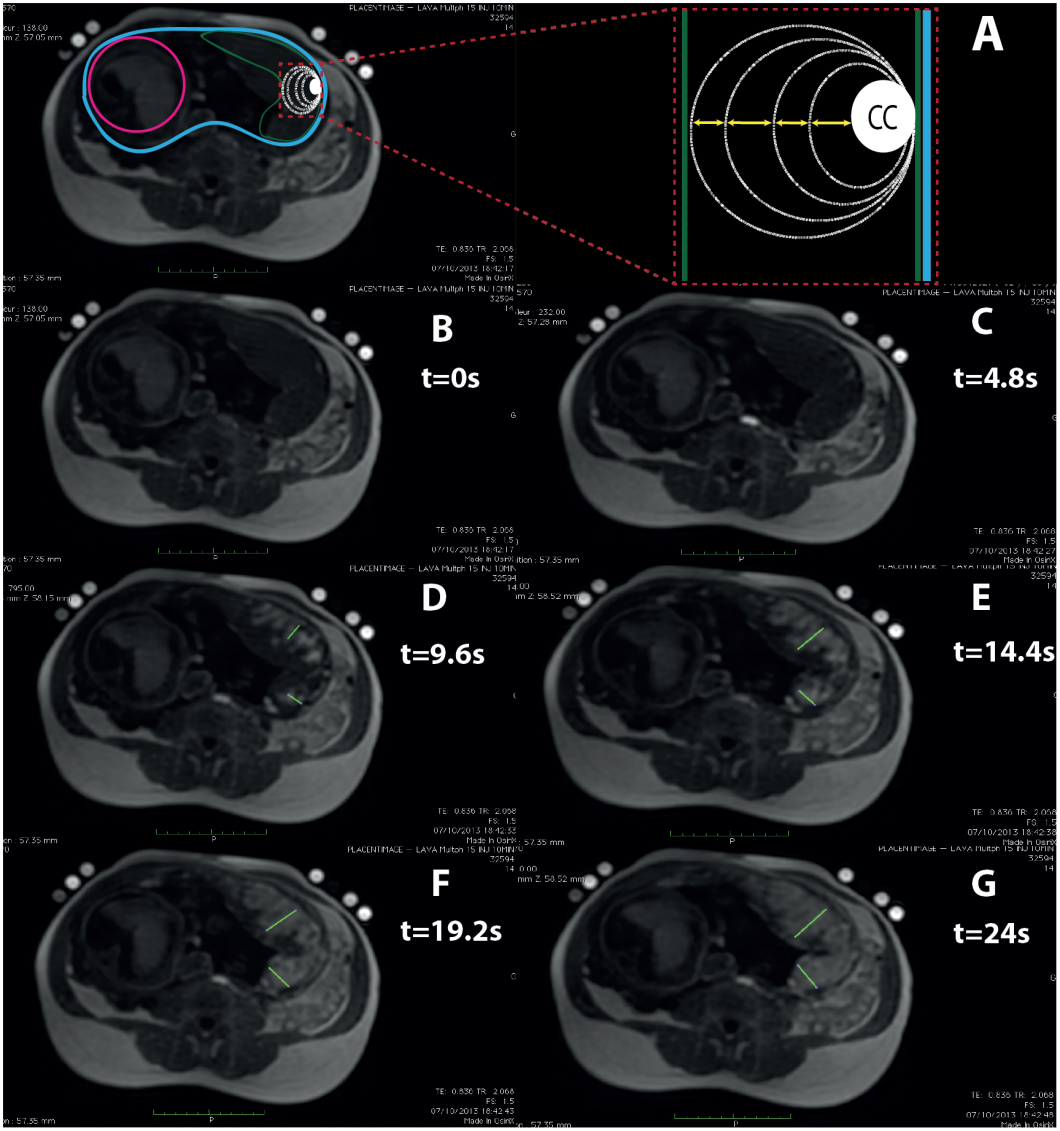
Dynamic placental MRI. A: Placenta (green line), uterus (blue line), fetus (pink line). The intraplacental progression of the contrast-enhanced maternal blood in the IVS was measured (yellow arrows) every Δt in a perpendicular direction between the basal plate and the chorionic plate. B-G: Progression of the gadolinium is measured every 4.8s, from the first MRI picture with gadolinium in the placenta (Fig 6D) in order to avoid calculating the velocity of the maternal blood in the central cavity (CC) of the placentone. The mean flow velocity of maternal blood measured in the IVS is 0.94±0.14 mm.s^-1^.
